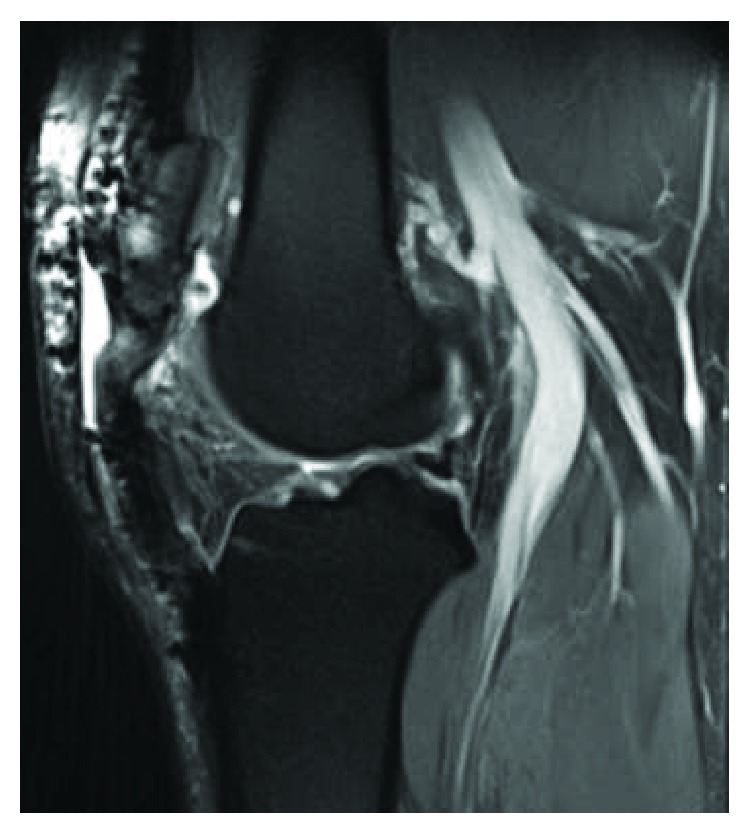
Postoperative MRI of the right knee at 18-month follow-up. Caton-Deschamps = 1.35.
